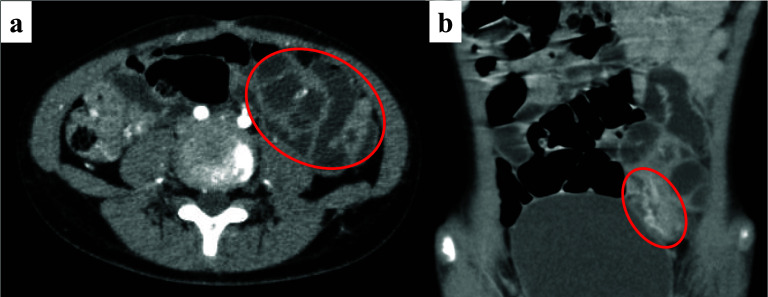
Enhanced CT findings. a Multilocular cystic lesion of 56 × 44 × 30 mm in size with partial calcification. b Dilated left ovarian vein
